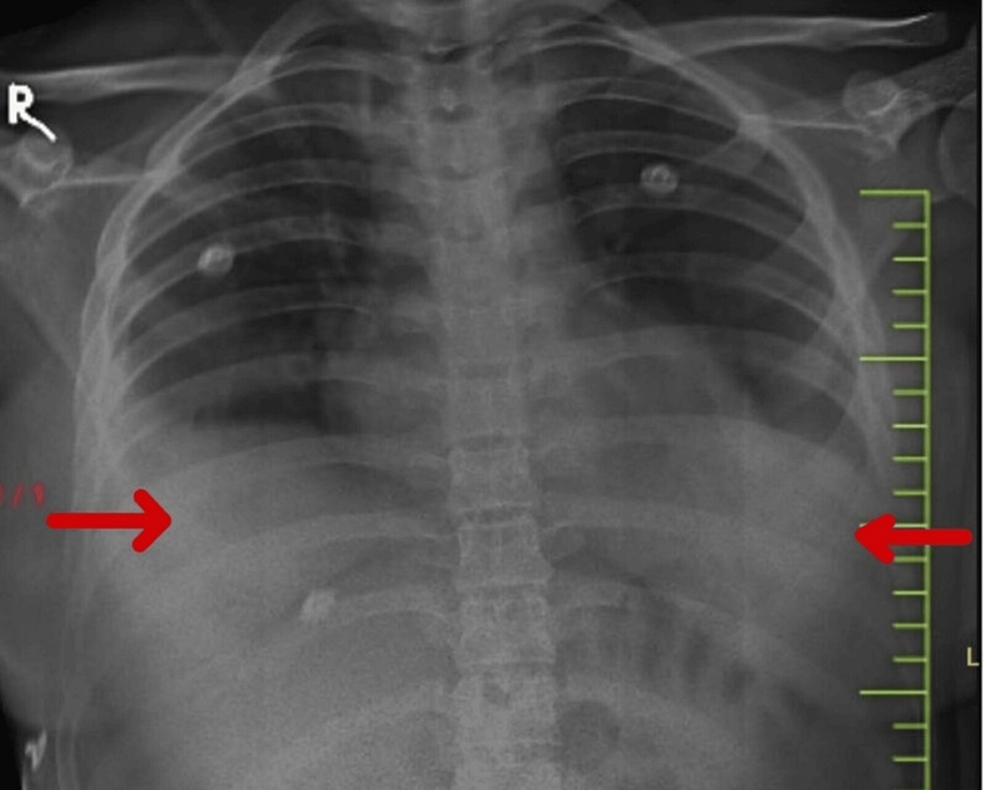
Chest X-ray showing blunting of the bilateral costophrenic angle.
